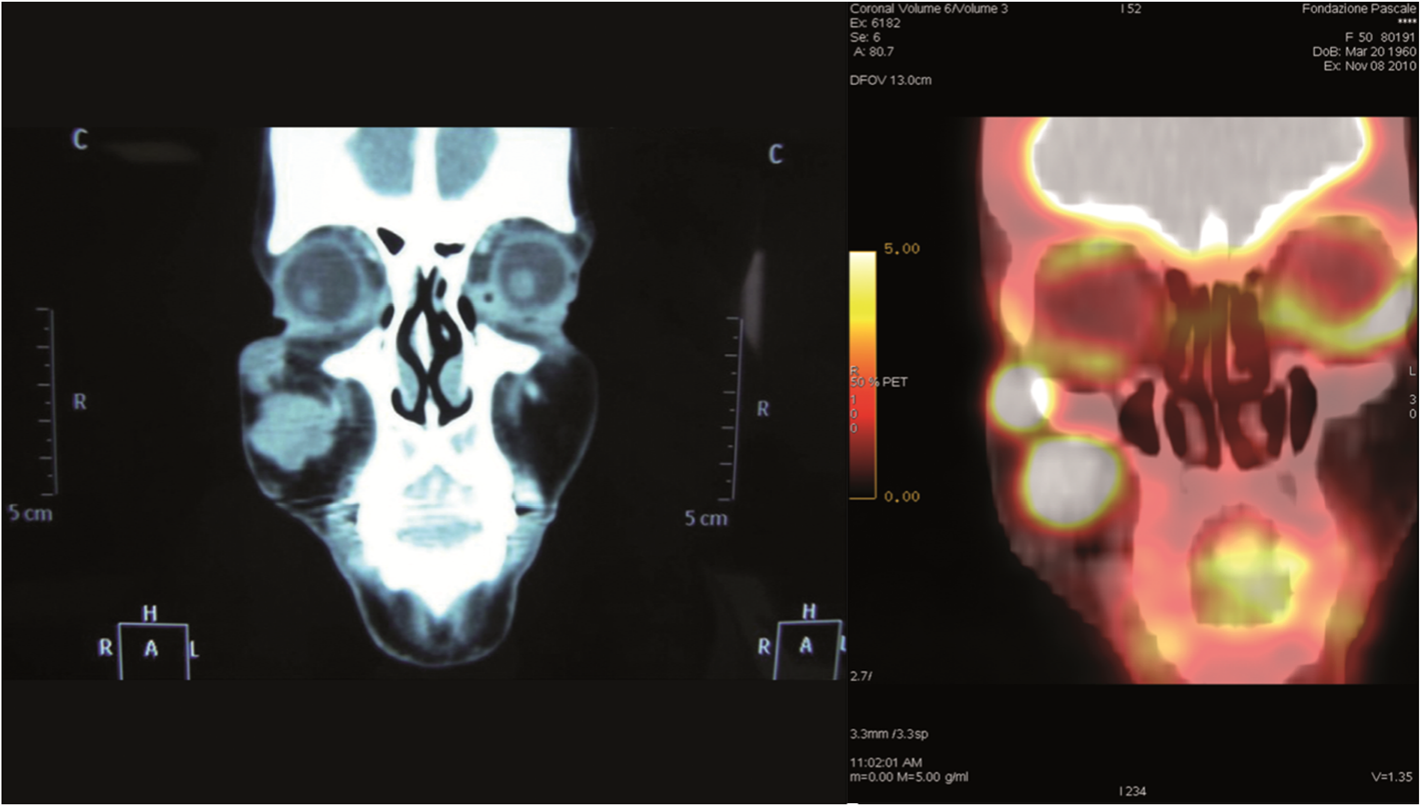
Pre-treatment CT scan with evidence of sandglass-shaped mass of the right cheek and FDG-PET scan with a SUVmax of 19.5.
